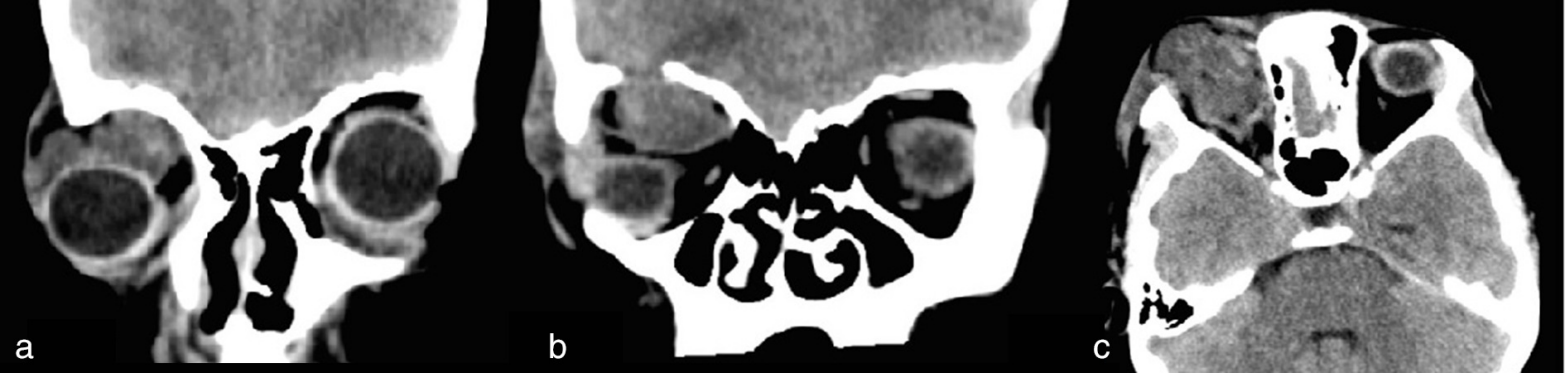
Coronal reformatted (a, b) and axial (c) CT soft tissue window images showing presence of a heterogeneous soft tissue density lesion in the right orbit protruding from a bone defect in the orbital roof. The thin dura is seen limiting the caudal extension of the herniated brain parenchyma.
